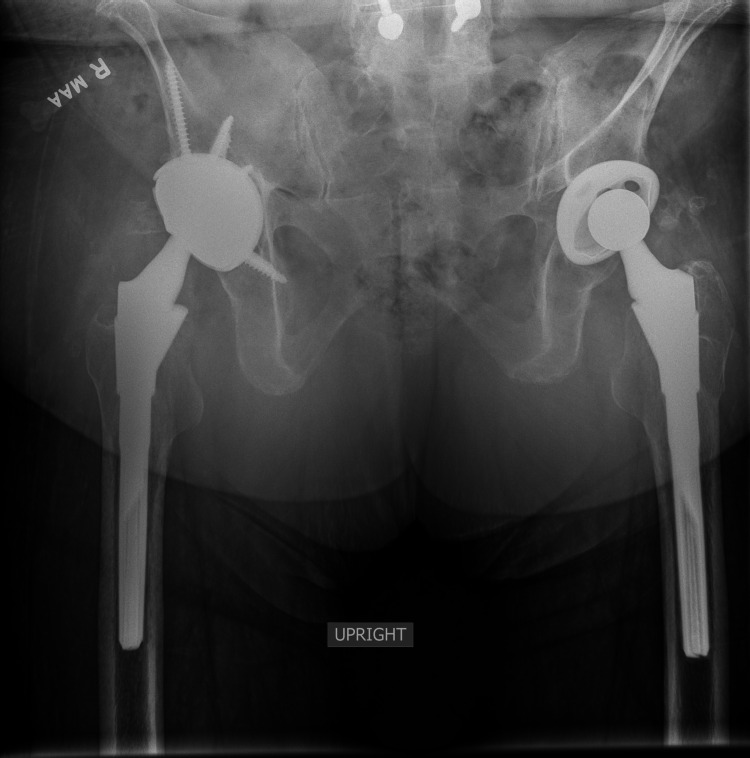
Three-month postoperative anterior-posterior pelvis radiograph The radiograph shows well-seated and aligned implants with no evidence of loosening or other component complications
